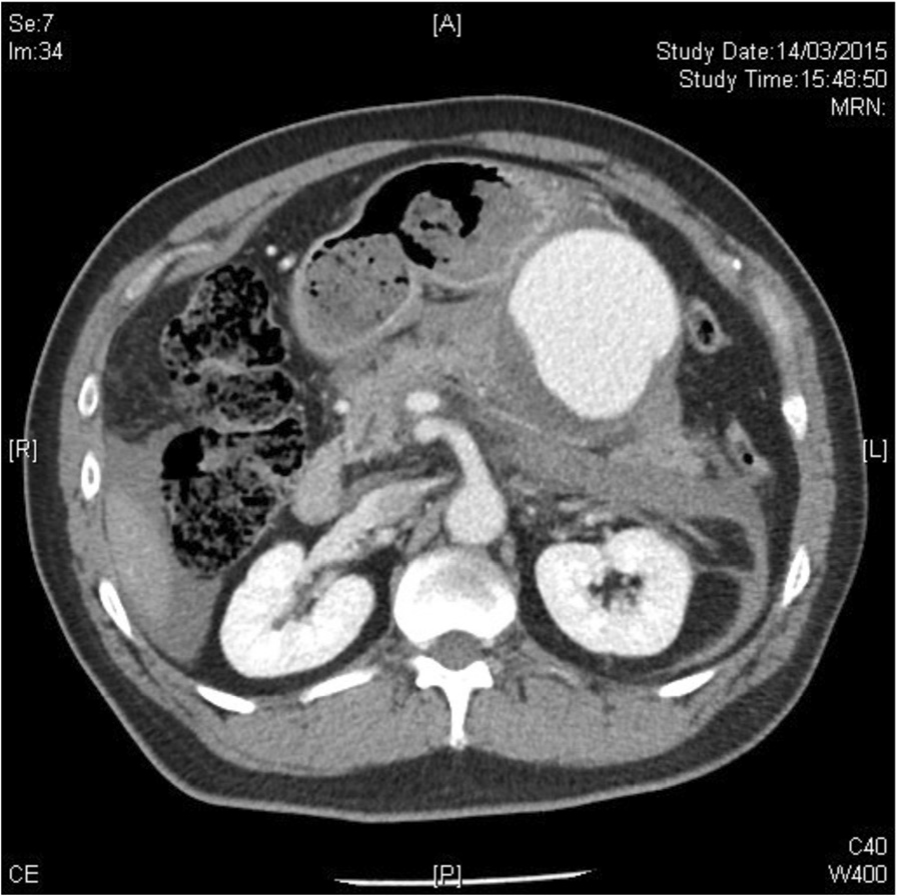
CT scan of the abdomen with IV contrast showing the splenic artery pseudoaneurysm in close proximity to the pancreas, with a partial thrombus and hyperdense hematoma. Hemoretroperitoneum and hemoperitoneum were again demonstrated
